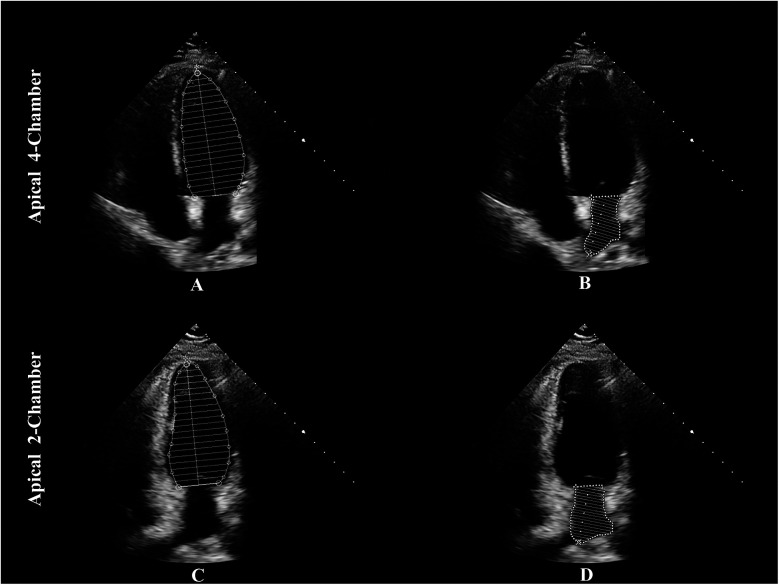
The method to assess LACI by echocardiography. LACI is calculated as the ratio of the minimum LA volume at end-diastole to the LV end-diastolic volume. The Simpson's biplane method is used to integrate measurements from apical two- and four-chamber views: panel (A) shows LVEDV in the apical 4-chamber view, panel (B) shows LAEDV in the apical 4-chamber view, panel (C) shows LVEDV in the apical 2-chamber view, and panel (D) shows LAEDV in the apical 2-chamber view. LACI, left atrioventricular coupling index; LA, left atrium; LV, left ventricle; LVEDV, left ventricular end-diastolic volume; LAEDV, left atrial end-diastolic volume.
